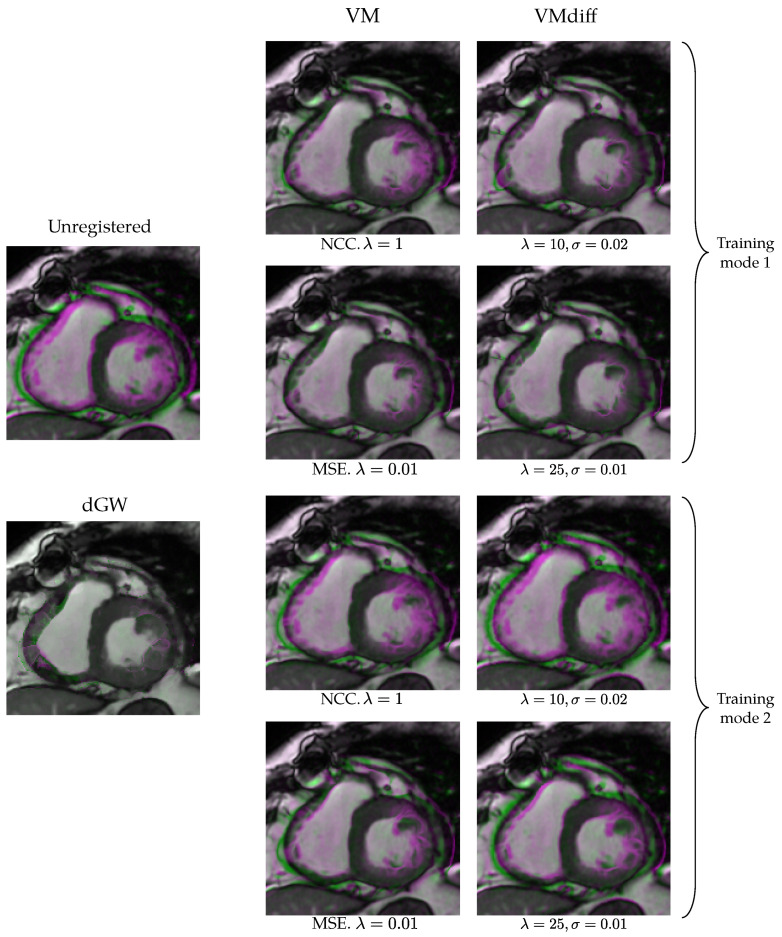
Differences between dGW and the eight VM implementation in registration quality. We show systole and a diastole superimposed frames for a slice of a patient in Database II. Leftmost column: unregistered cine cardiac MRI (up), dGW registration (down). Middle column: the four VM-2 implementations. Rightmost column: the four VMdiff implementations. Pink: registered systole with higher intensity. Green: registered diastole with higher intensity.
